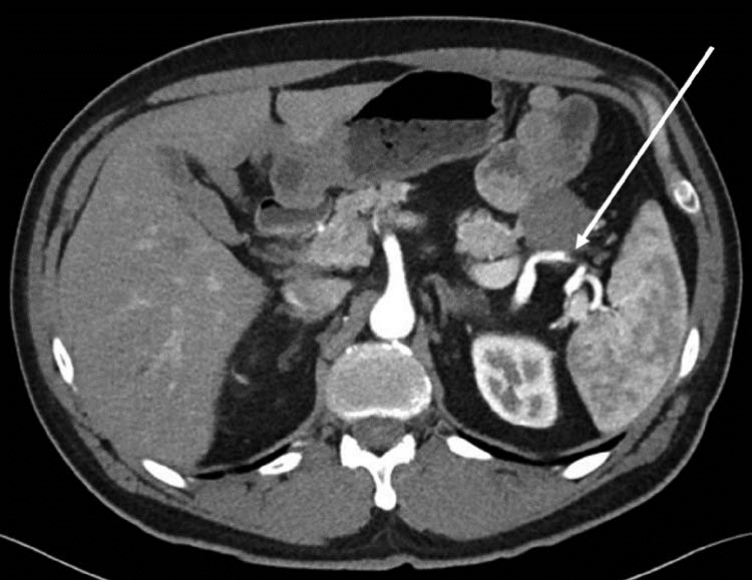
Axial CT scan of abdomen, selected image from a pancreatic protocol. Early arterial phase during which there is opacification of the arterial structure (arrow).
